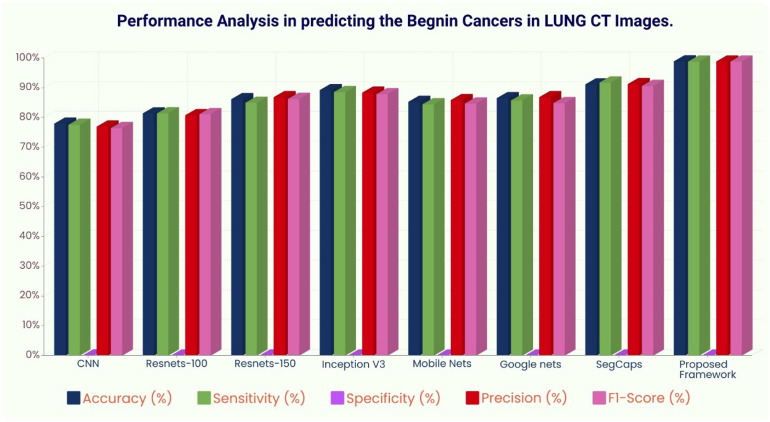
Performance analysis in predicting benign tissue in lung CT images.
